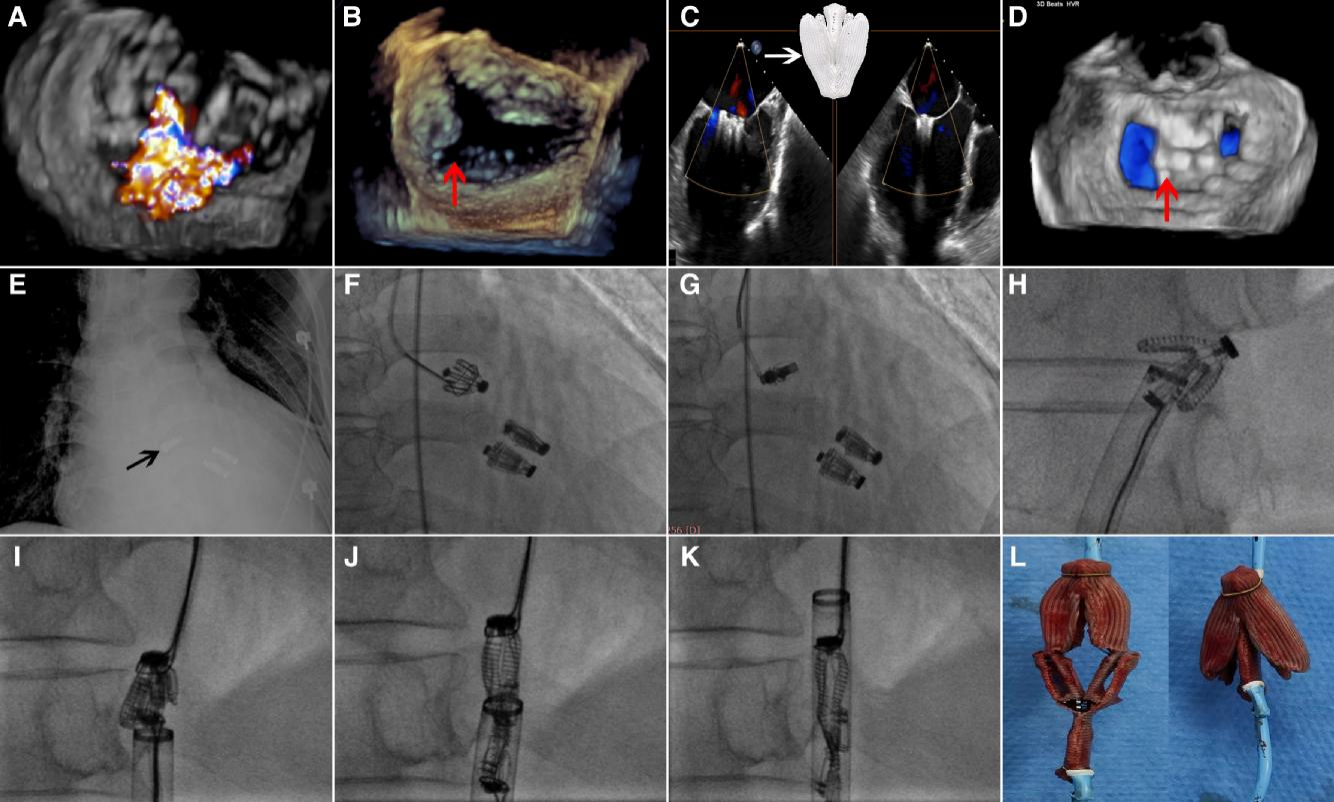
Procedural steps of percutaneous retrieval of embolized ‘Pascal-like’ clip device. (*A* and *B*) 4+ FMR was noted with big indentation at P1–2 area (red arrow); (*C* and *D*) Three Clip (white arrow) was implanted with one clip locating at indentation area (red arrow). (*E*) Bedside X-ray confirmed clip embolization (black arrow). (*F-H*) The embolized clip was pulled back into the descending aorta by gooseneck snare. (*I-K*) Using two gooseneck snares technique, clip was successfully removed through 20 F sheath. (*L*) *In vitro* demonstration of the removed ‘Pascal-like’ clip.
